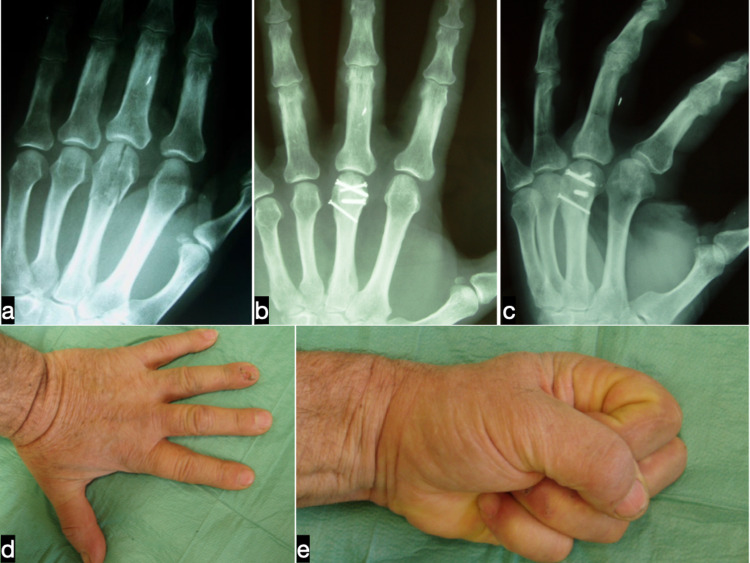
Preoperative (a) anteroposterior radiograph of a 64-year old male carpenter who sustained an open intra-articular fracture of the third metacarpal head of his non-dominant hand. The fracture was treated with four titanium mini screws in an effort to restore the joint line. Radiographic (b,c) and clinical (d,e) outcomes seven years postoperatively.
